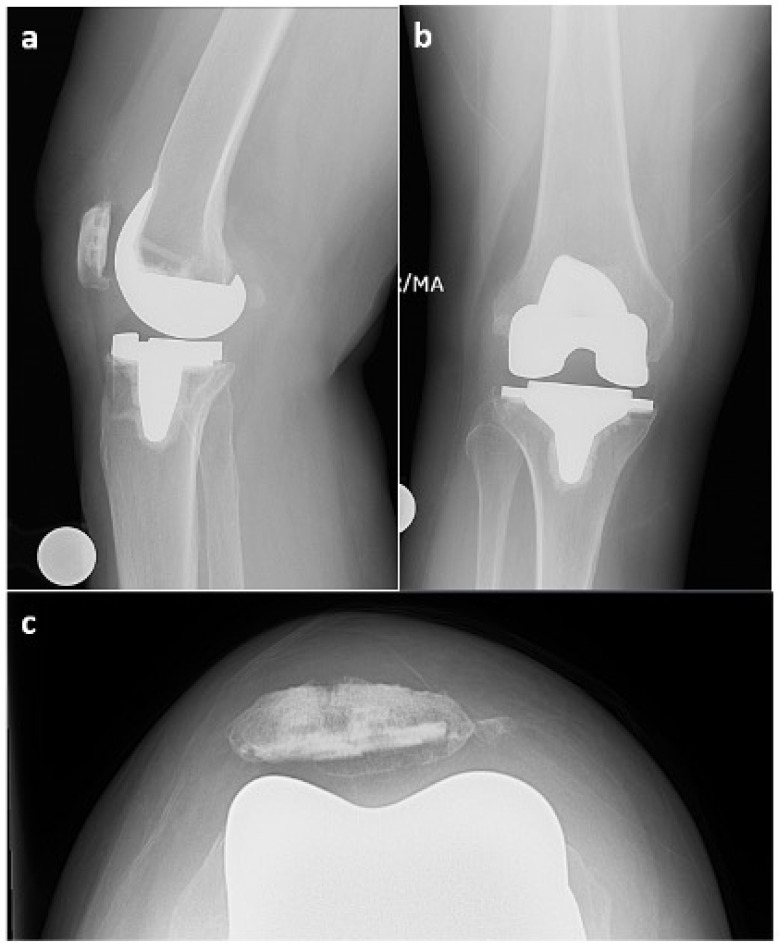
X-rays from 9 September 2022 ((a)—lateral view, (b)—anterior–posterior view, (c)—Merchant view). Right total knee arthroplasty (TKA) was reidentified with components in anatomic alignment without fracture.
